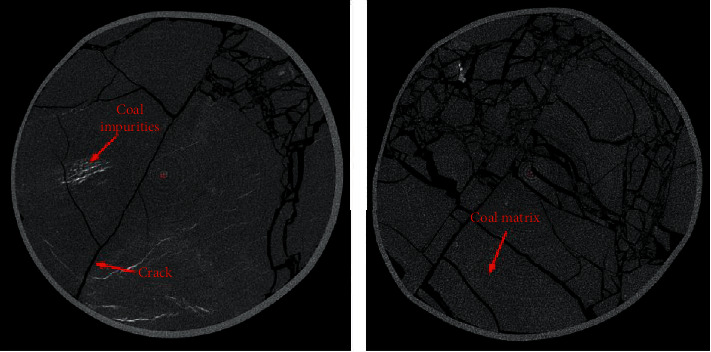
CT image samples of coal crack.
